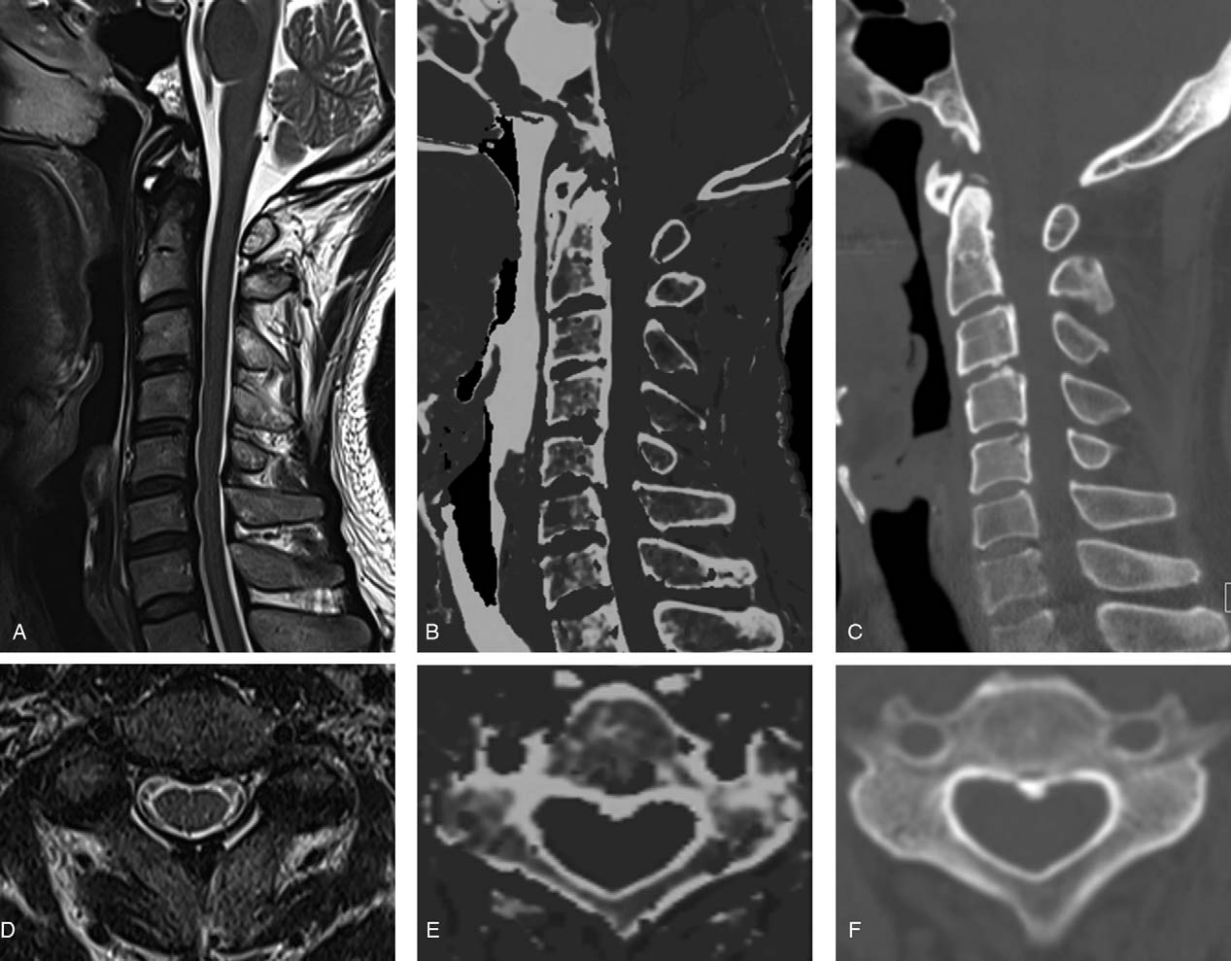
A 54-year-old-man with cervical OPLL.A, D. In session 1 (conventional MRI alone), two radiologists determined that there was thickening of cervical PLL but no OPLL at T2-weighted images. B, E. In session 2 (MRI + MRCT), two radiologists evaluated the presence of an OPLL at the C2–C5 level with the addition of MRCT to conventional MRI images. C, F. In session 3 (conventional CT), cervical OPLL was noted at the C2–C5 level, as the reference standard. MRCT = magnetic resonance-generated synthetic computed tomography, MRI = magnetic resonance imaging, OPLL = ossification of posterior longitudinal ligament, PLL = posterior longitudinal ligament.
